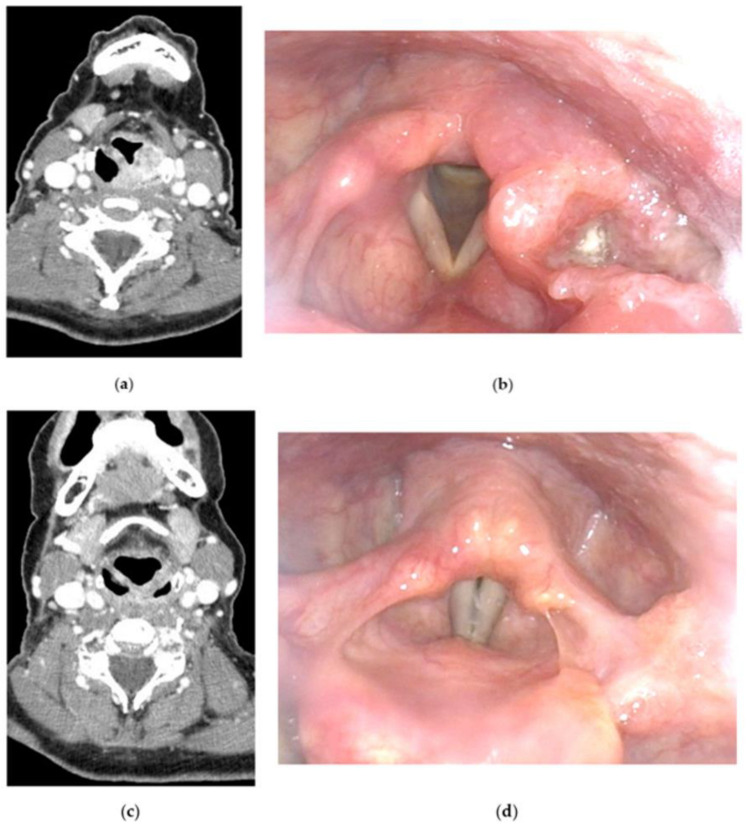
Induction chemotherapy (ICT)-based larynx preservation (LP) program for a 62-year-old female patient with a T3N0 hypopharyngeal carcinoma: (a) initial CT scan of the tumor; (b) initial nasofibroscopy showing a T3 tumor invading the left pyriform sinus, and the aryepiglottic fold; (c) post-ICT CT scan showing a near-complete response to ICT (2 cycles of TPF: docetaxel, cisplatin and 5-fluorouracil); (d) post-ICT nasofibroscopy showing a near-complete response to ICT with larynx mobility recovery.
